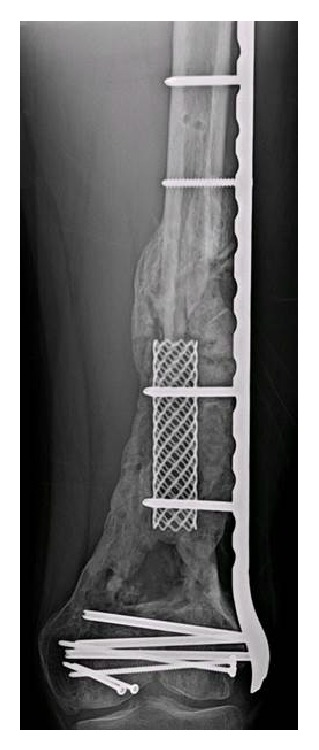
Radiograph demonstrating remarkable regrowth of new bone surrounding the endosteal titanium implant at 8 months post-procedure. Note the contour of the new bone, formed within the pseudocapsule which resulted from the initial antibiotic cement spacer used in the first stage of the Masquelet technique.
